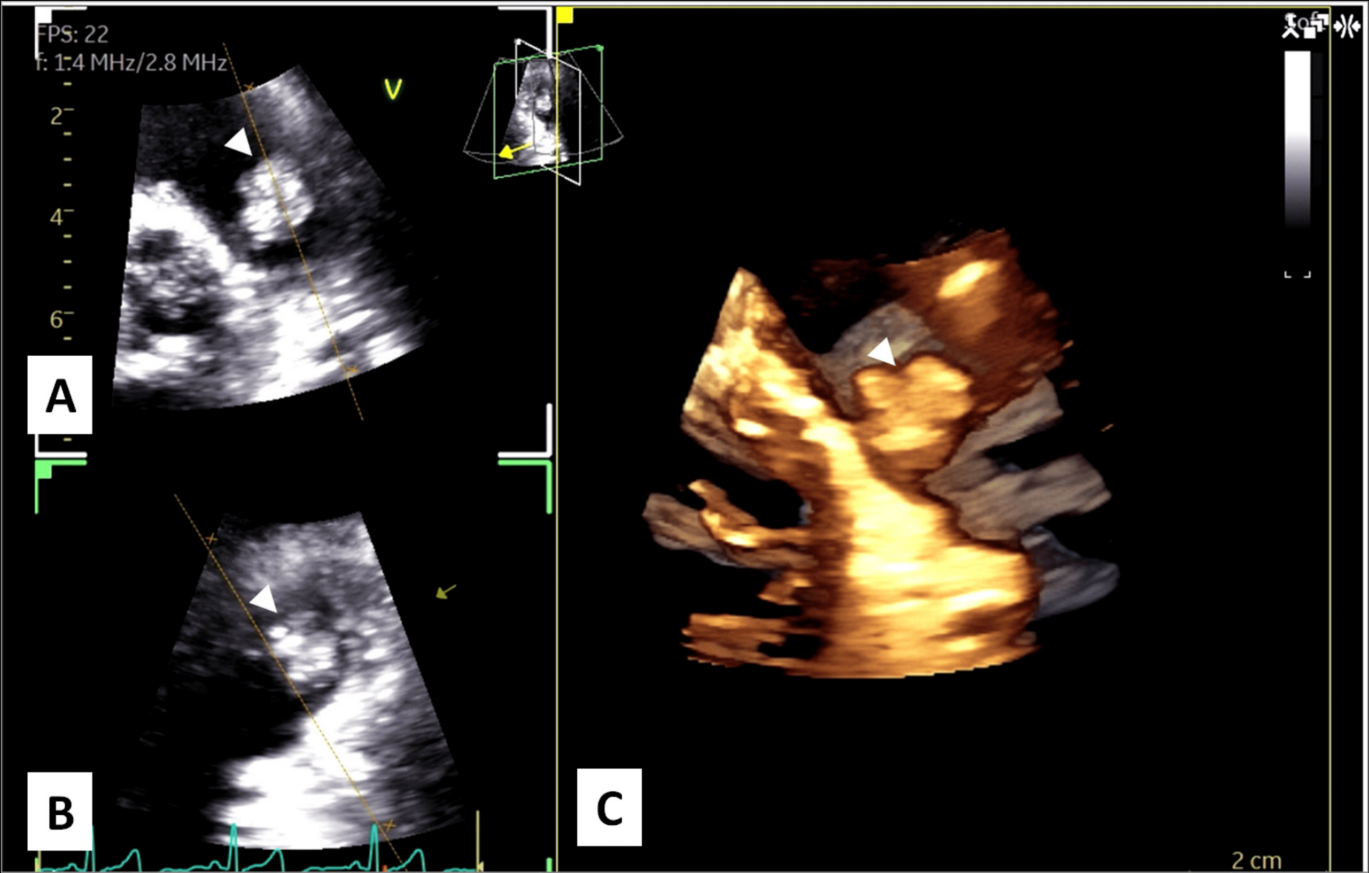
Transthoracic Echocardiography With Two-Dimensional and Three-Dimensional Imaging (A) and (B) demonstrate two-dimensional transthoracic echocardiographic views showing a mobile, heterogeneous mass (arrowheads) arising from the pulmonary valve and protruding into the right ventricular outflow tract during the cardiac cycle, while (C) demonstrates three-dimensional transthoracic echocardiographic reconstruction providing detailed spatial visualization of the lobulated mass attached (arrowheads) to the pulmonary valve, allowing improved assessment of its morphology and valve involvement.
